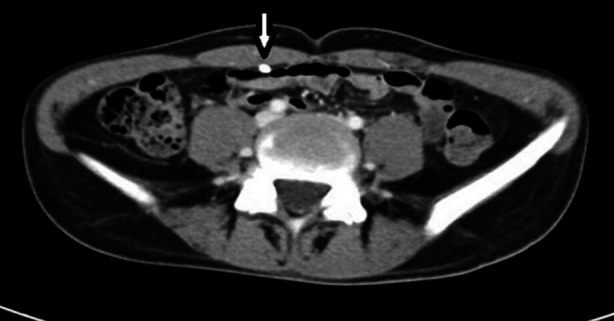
Contrast-enhanced CT abdomen scan axial image demonstrates the normal right iliac fossa two months before the patient most recent hospitalization. The white arrow points to the intraperitoneal catheter of the right-sided VPS.
